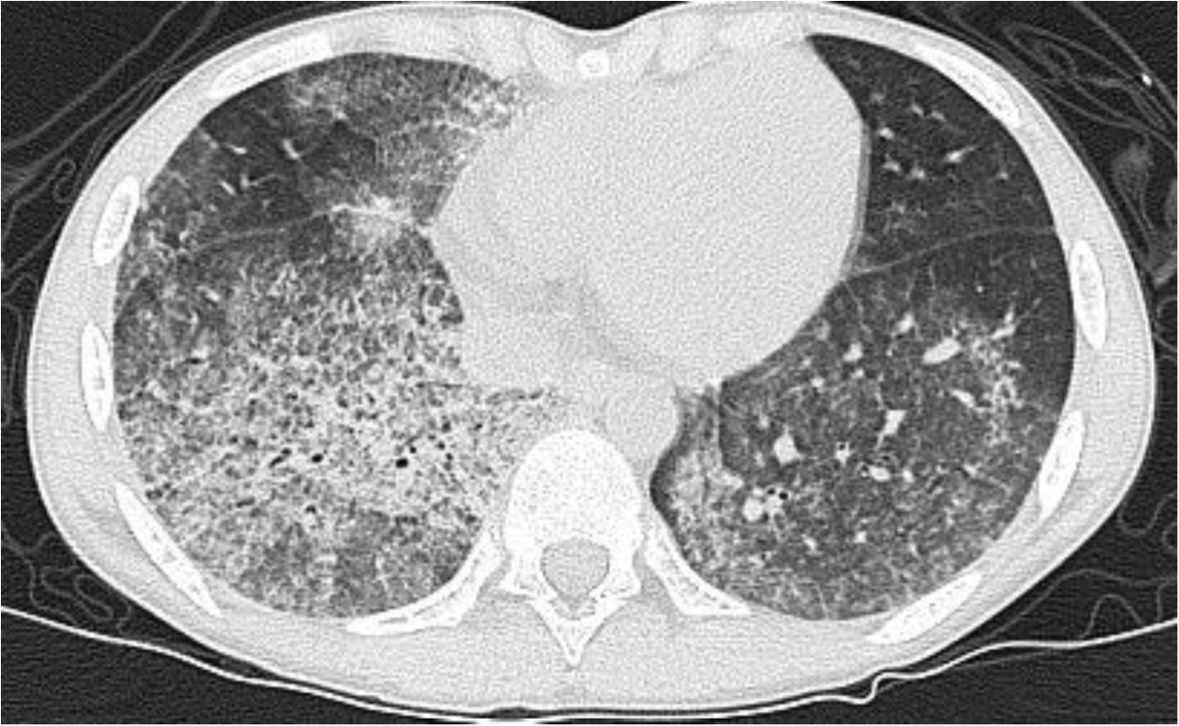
High resolution computed tomography with contrast medium showed patchy inhomogeneous opacities in bilateral lungs, more on the right side than the left side
